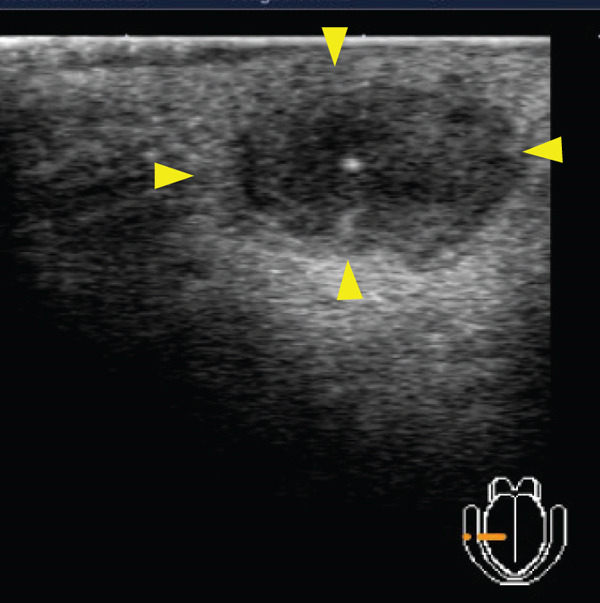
Ultrasonographic image at first visit. The image revealed a punctate hyperechoic focus within a hypoechoic lesion.
